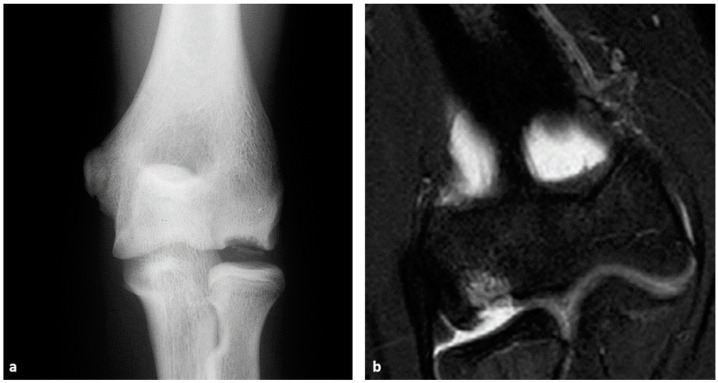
OCD lesions in different patients: (a) Anteroposterior X-ray view of the elbow—an irregular capitellum with a radiolucent defect with sclerotic contours and fragmentation of the articular surface; (b) PD-weighted fat-suppressed coronal MR image—full-thickness chondral defect of the capitellum with edema of the subchondral bone.
